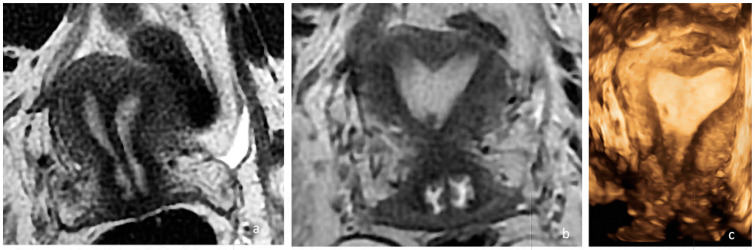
Preoperative and postoperative examination of case 2. (a) The preoperative MRI T2, WI, confirmed the presence of a Müllerian anomaly and identified it as a complete septate uterus, duplicated cervix, and longitudinal vaginal septum (Class U2bC2V1 of the ESHRE/ESGE classification). A complete septum with a thickness of 3 mm and a length of approximately 6 cm (measured from the intercolonial line) and 7.5 cm (measured from the level of the external contour of the uterus) was identified. (b) The postoperative MRI at 2 months confirmed the partial persistence of the septum in the supracervical region with the persistence of a slight indentation at the fundal level appearance of an arched uterus. (c) Three-dimensional ultrasonography performed 5 months postoperatively in which a normal uterine cavity was observed.
